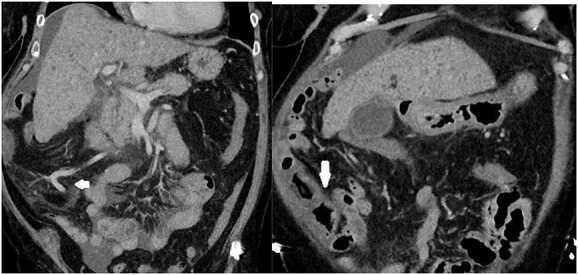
Images depicting a single slice of the CT Abdomen/Pelvis with contrast completed on admission. Arrows pointing to subtly dilated mesenteric vessels, as well as thickened bowel affected by PHC, is depicted in Fig. 2.
